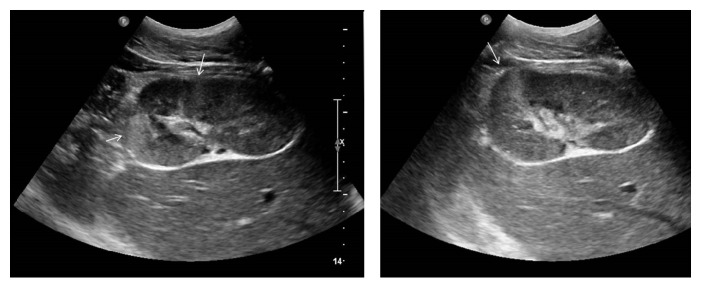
Findings: grey scale ultrasound image of right kidney showing ill-defined echogenic areas at lower pole of right kidney.
